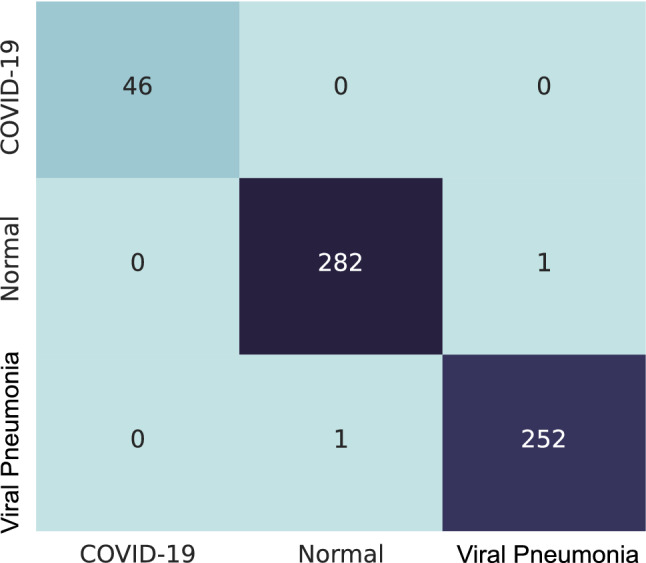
Confusion matrix for COVID-19 Radiography Database
